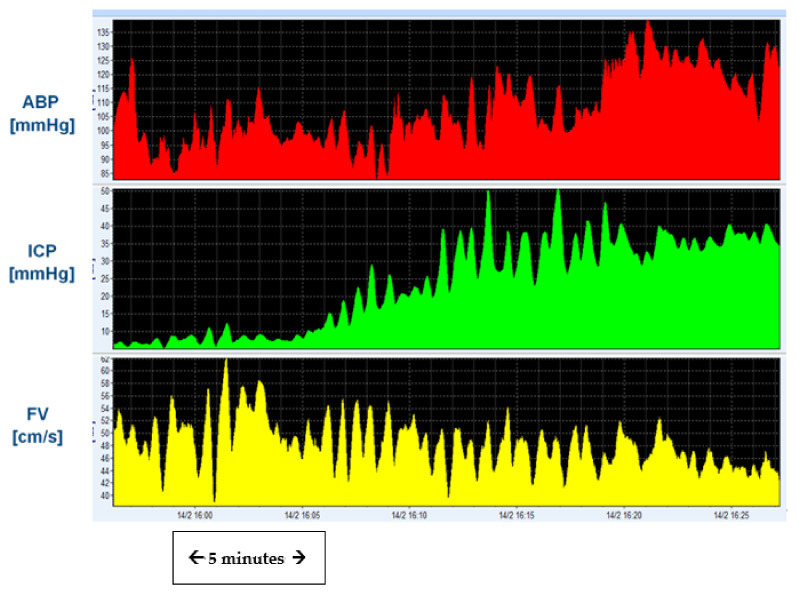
Slow waves of ICP, arterial blood pressure and cerebral blood flow velocity. Simultaneous recording of mean arterial blood pressure (ABP), ICP, and transcranial doppler derived flow velocity (FV) recording through the middle cerebral artery. Note the similarities in frequency and synchronicity in slow waves of FV and ICP. Cerebral autoregulation indices can be calculated from both ICP and ABP, as well as FV and CPP (ABP–ICP).
